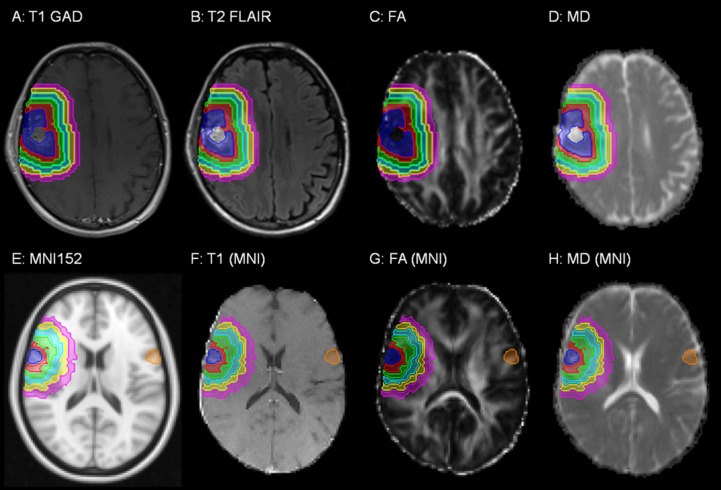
Magnetic resonance images (MRI) obtained for a sample patient. The (A) T1-weighted MRI with gadolinium contrast enhancement, (B) T2-weighted FLAIR MRI, (C) fractional anisotropy (FA), (D) mean diffusivity (MD), (E) the MNI 152 symmetric standard space image, (F) T1-weighted MRI registered to the symmetric standard space image, (G) FA image registered to the symmetric standard space image, and (H) MD image registered to the symmetric standard space image are shown. Regions of interest shown are the GTV (blue), peritumoral shells 0-5 mm (red), 5-10 mm (green), 10-15 mm (cyan), 15-20 mm (yellow), and 20-25 mm (magenta) outside the GTV, and GTV mirrored in the left-right direction (orange).
